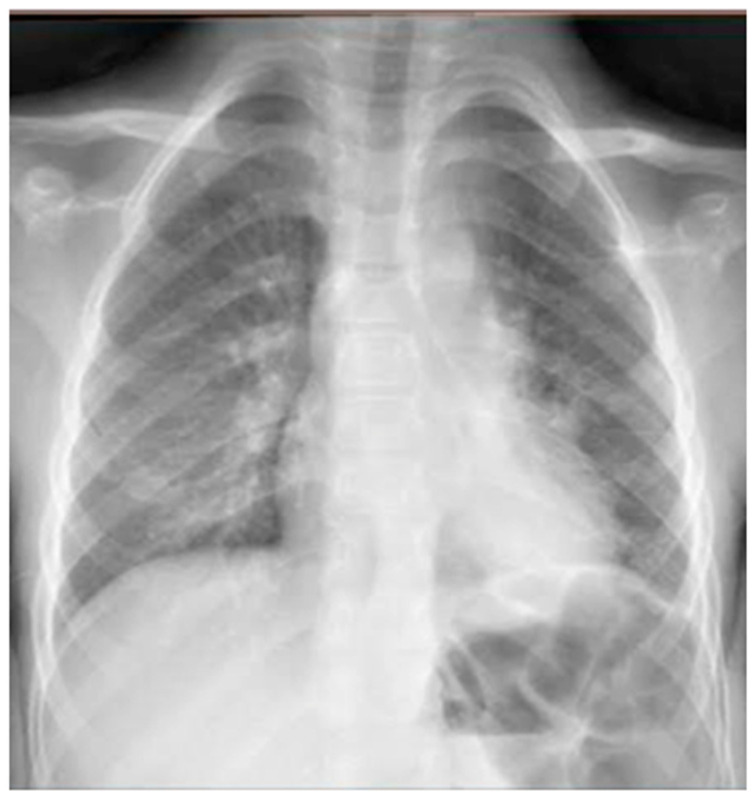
Chest radiography shows, on the left inferior lobe, non-specific areas of reduced transparency, associated with obliteration of the left lateral costophrenic sinus. It shows further subtle parenchymal hypodiaphania on the right mid-basal area.
